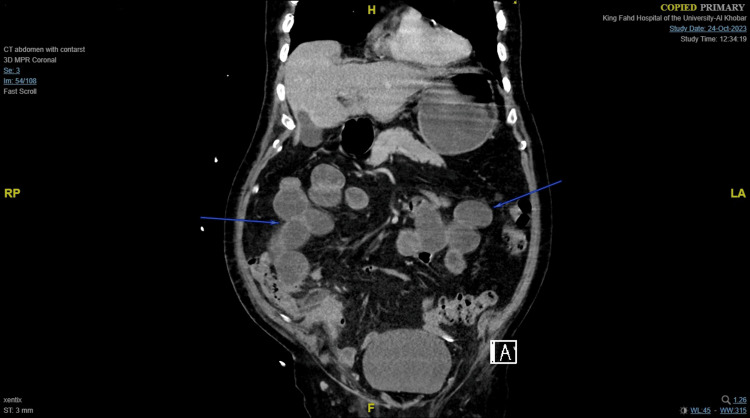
Follow-up contrast-enhanced CT scan of the abdomen on the sixth postoperative day showing fluid-filled dilated jejunal and ileal loops (reaching 4.8 cm) with no transition zone.
